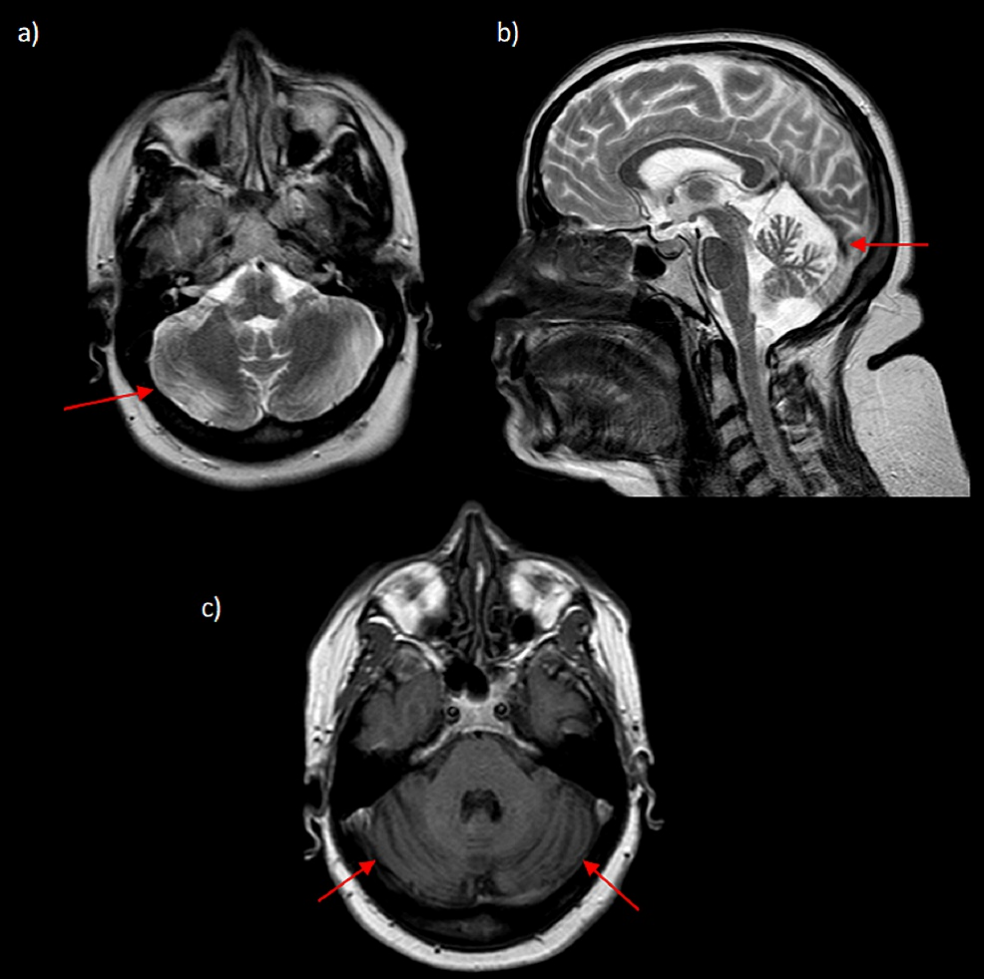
Brain MRI of Patient 1 a) Axial T2 sequence showing cerebellar atrophy; b) Sagittal T2 sequence showing cerebellar atrophy and microcephaly; c) axial T1 sequence with evident cerebellar atrophy
